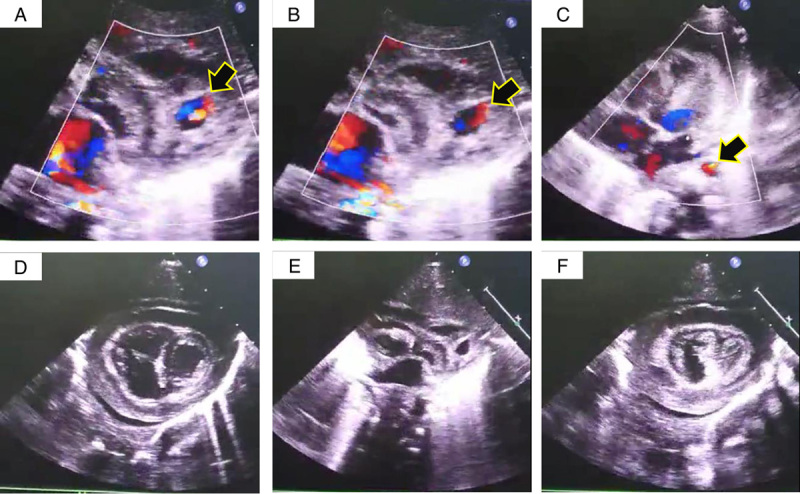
Echocardiography showing the left ventricular diverticulum (arrows in A–C), and hemopericardium with blood clots (in D–F).
